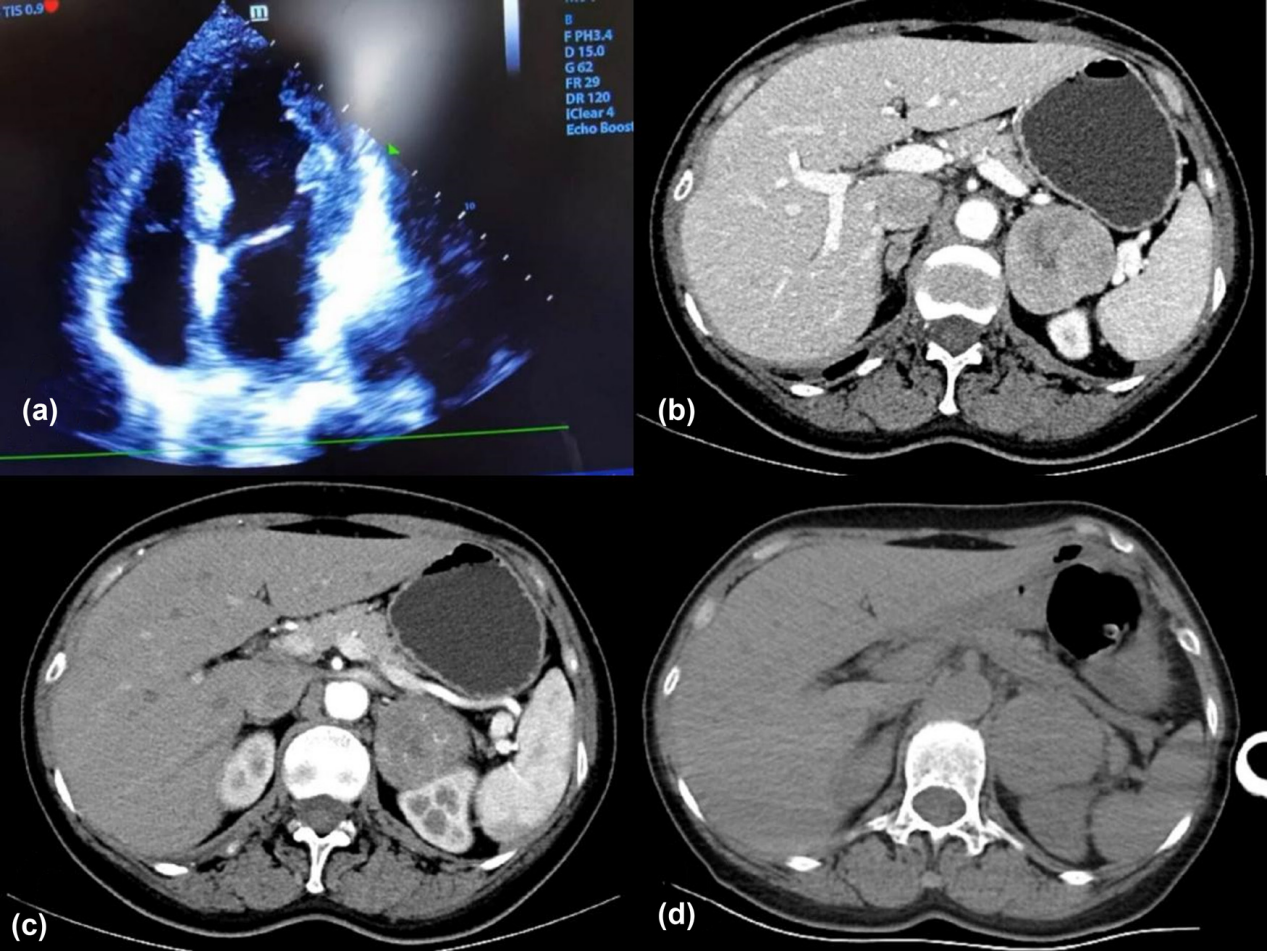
Results of echocardiography and adrenal gland CT scan. Echocardiography showed apical bulbous motion with an EF of 63% (a). CT scan of the adrenal gland revealed bilateral adrenal mass, with the left adrenal mass being larger in size (b–d).
